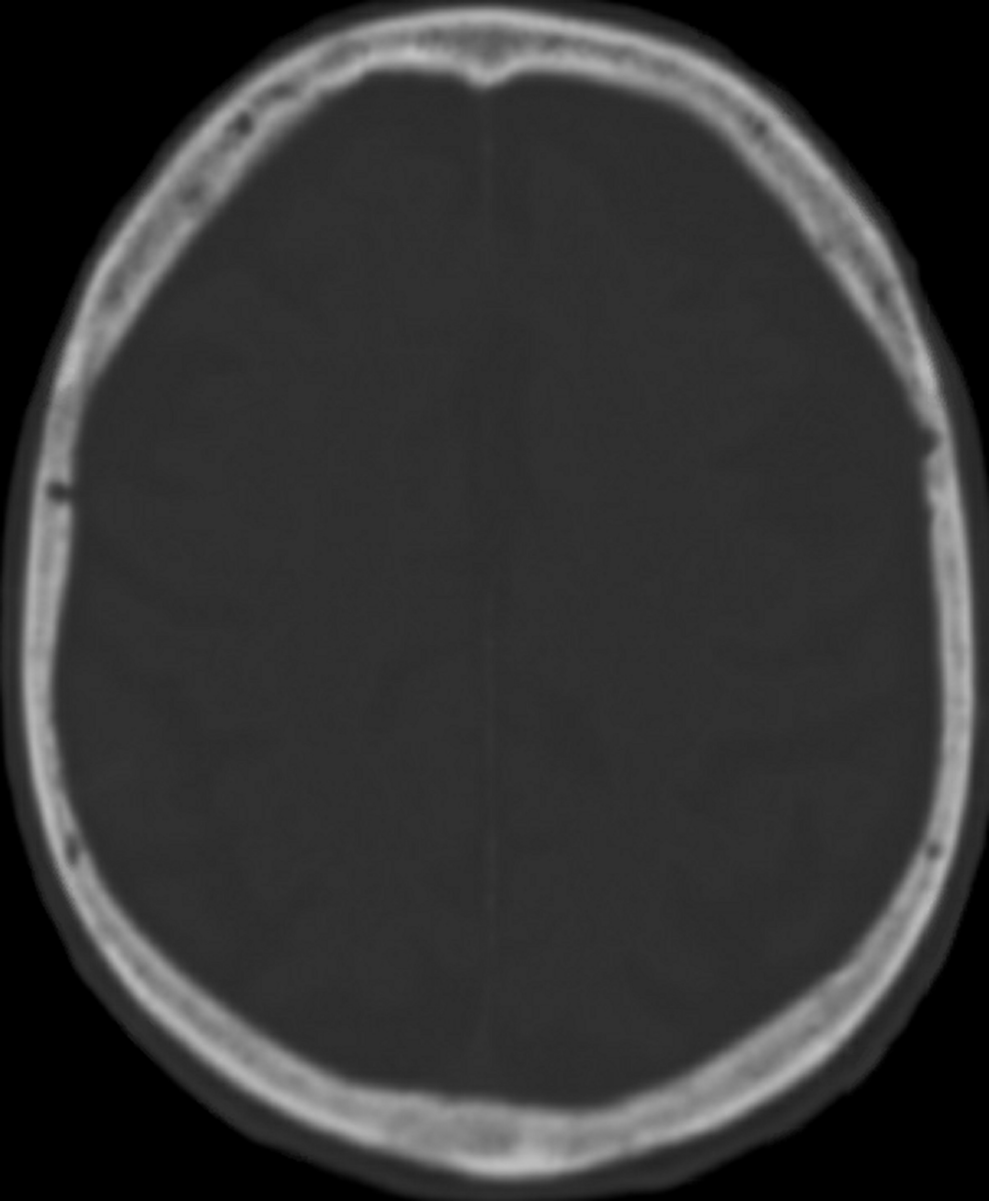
Axial view of a computed tomography scan of the calvaria, bone window, no evidence of depressed skull fracture.
